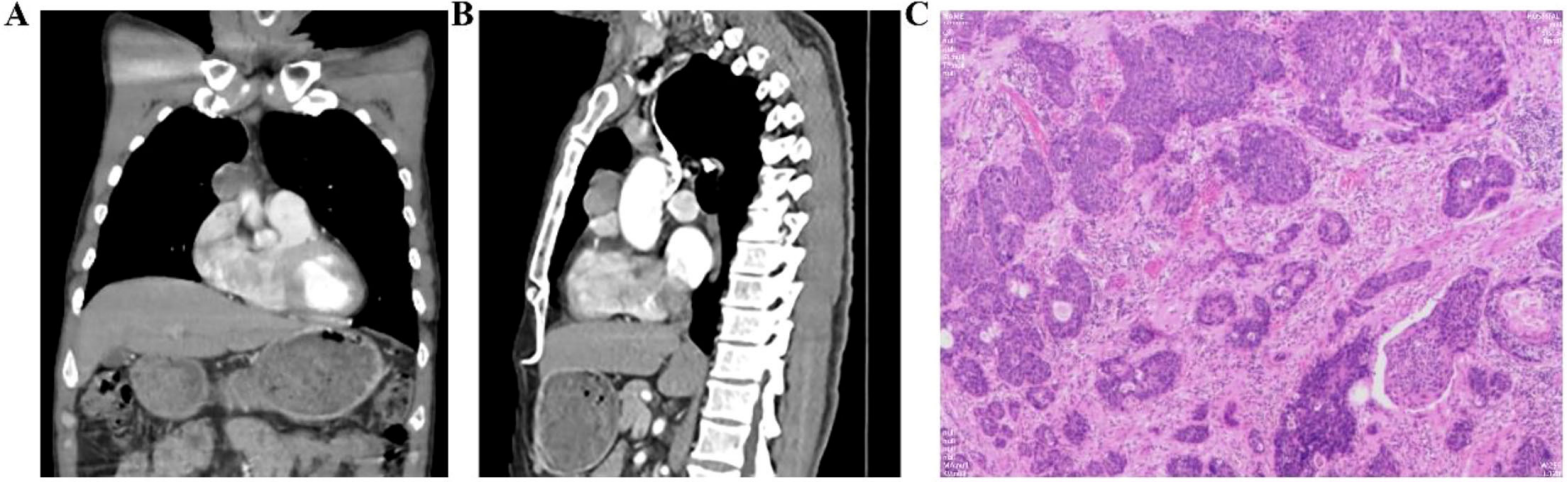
Enhanced chest CT showing mediastinal masses and postoperative pathological results of the esophagus. (A, B) mediastinal masses; (C) The pathological result of the esophagus.
